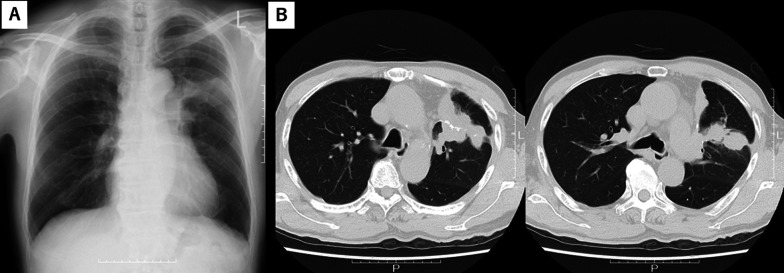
Radiographic findings for case 1. A Chest radiography revealed an abnormal shadow in the left upper region. B Computed tomography showing a granulomatous tumor of approximately 3 cm in diameter
